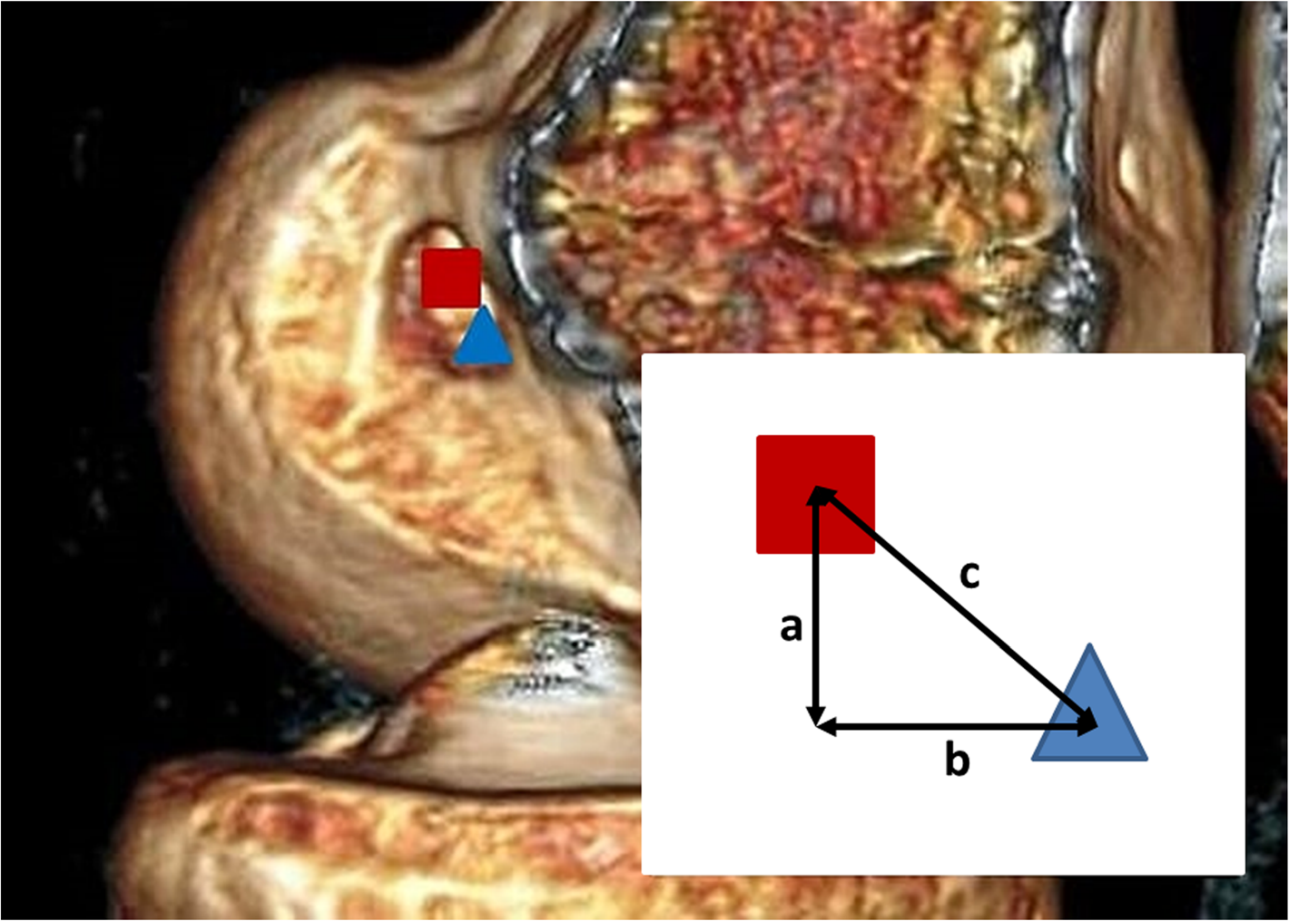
Calculation of difference between centre of tunnel aperture (AC; red square) and anatomical centre of anteromedial bundle (TC; blue triangle) shown on three-dimensional computed tomography reconstructed image; measurements a and b parallel and perpendicular (respectively) to Blumensaat’s line (on expanded view, distance c = √(a^2^ + b^2^))
